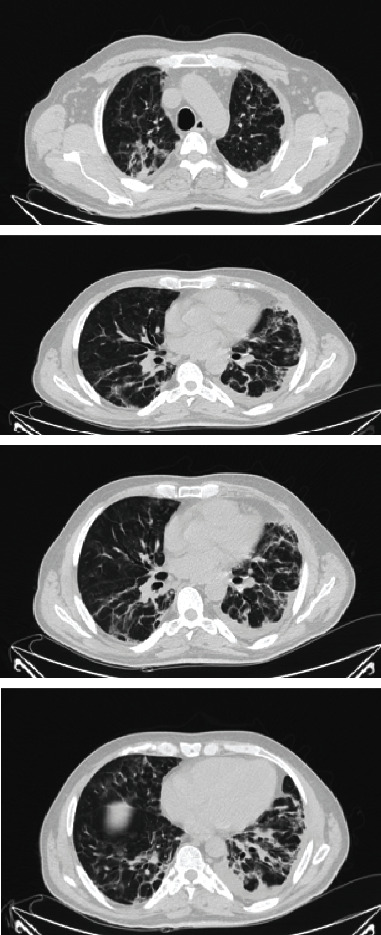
Chest CT showing permanent damage to the lungs by the coronavirus.
